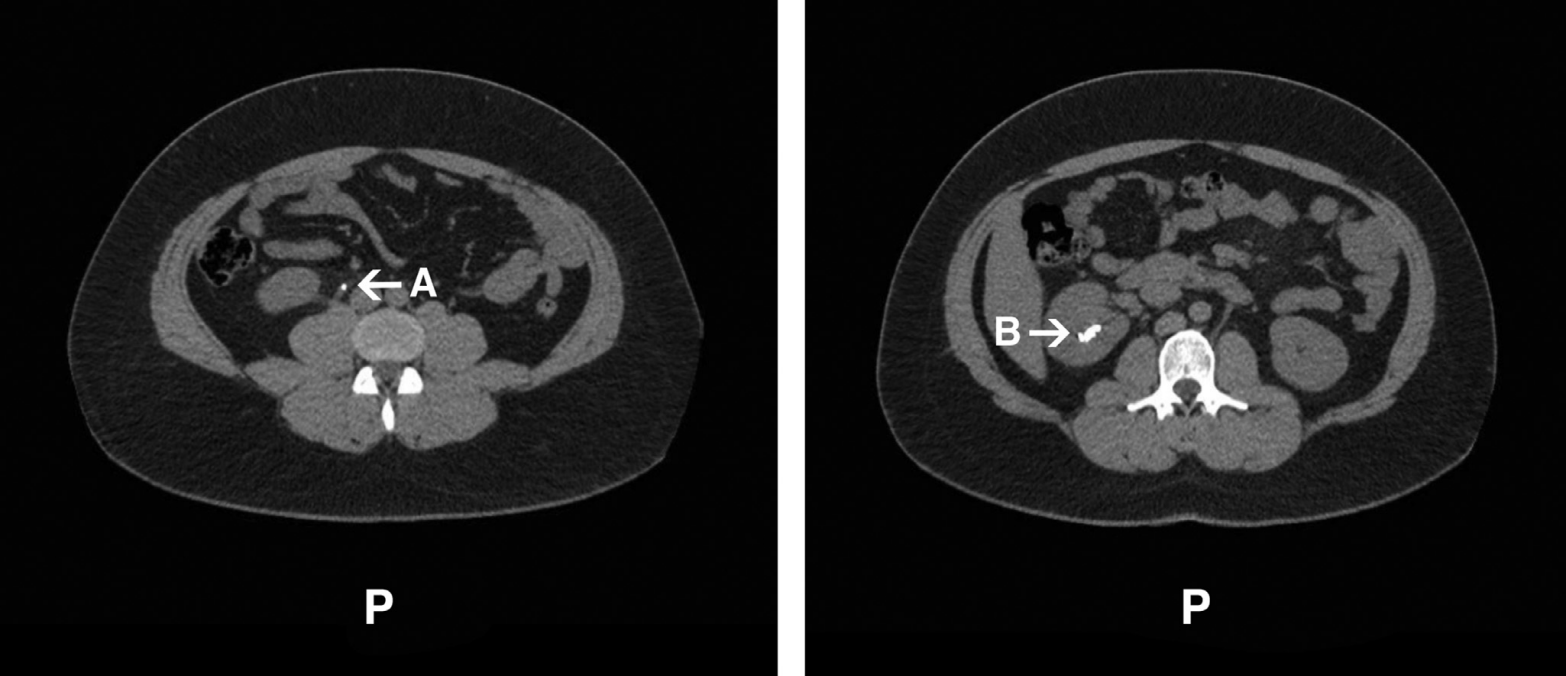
Non-contrast CT of kidneys, ureter and bladder. Transverse non-contrast CT scan of kidneys, ureter and bladder, confirming stent position, patency and successful clearance of a partially obstructive right ureteric calculus. Left: confirmed position of ureteric double-J stent in situ (A). Right: a clinically insignificant nonobstructive 8 mm caliceal calculus is seen in the upper polar region of right kidney (B).
